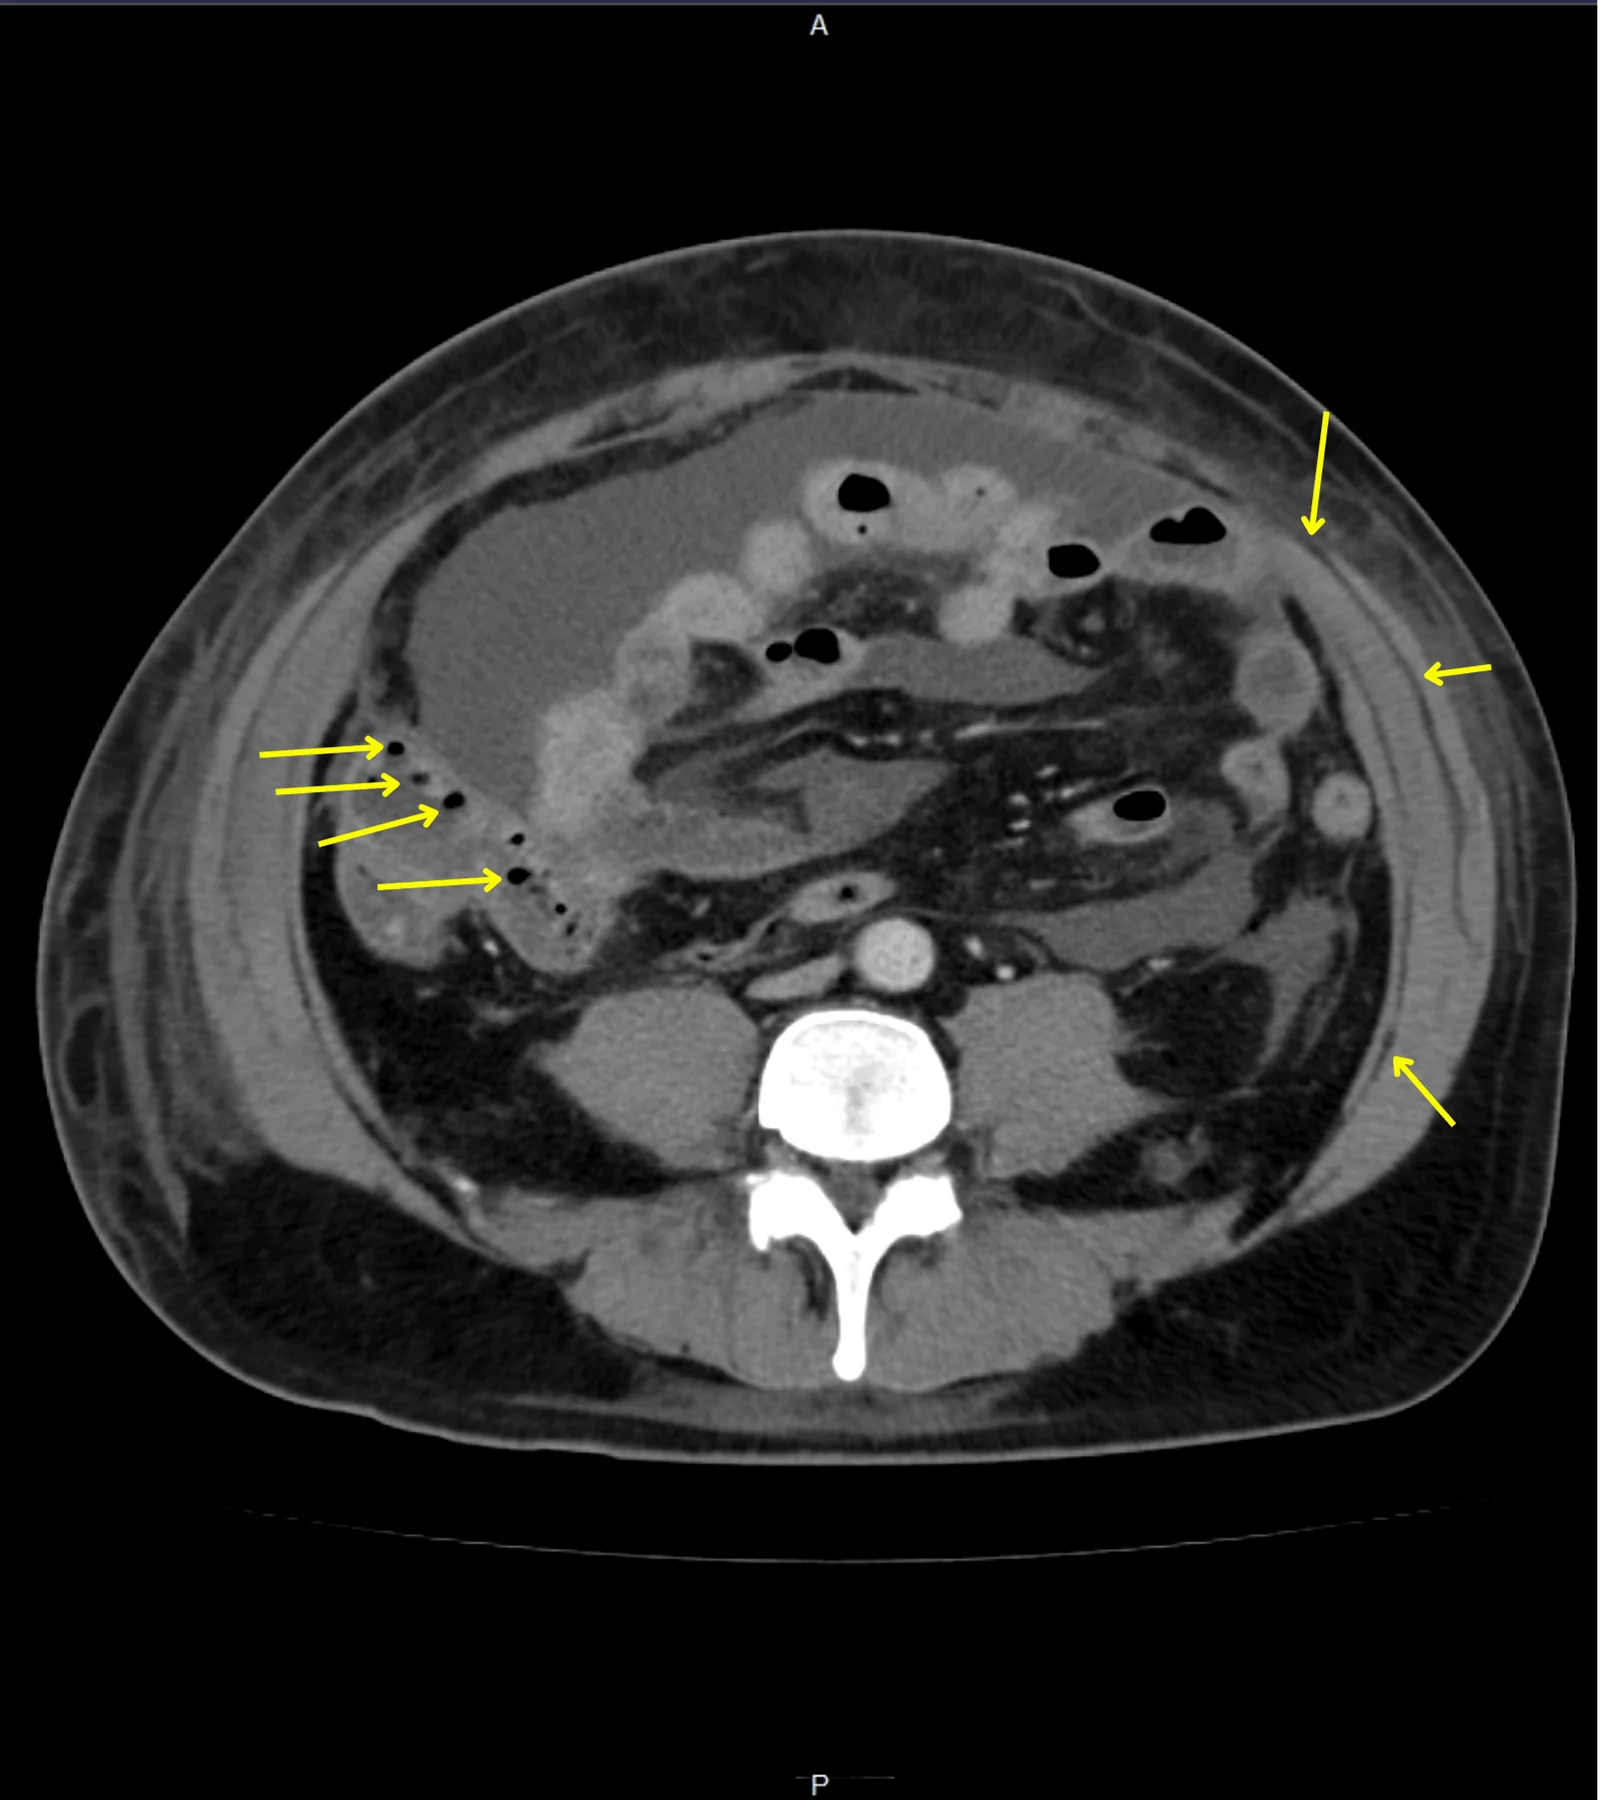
Axial contrast‑enhanced CT of the abdomen demonstrating diffuse intraperitoneal free fluid Arrows along the right abdominal wall highlight low‑attenuation fluid layering in the left paracolic gutter and anterior abdomen, while the left cluster of arrows indicates additional dependent free fluid outlining small‑bowel loops, consistent with the reported intraperitoneal and mesenteric fluid collections following recent cholecystectomy.
